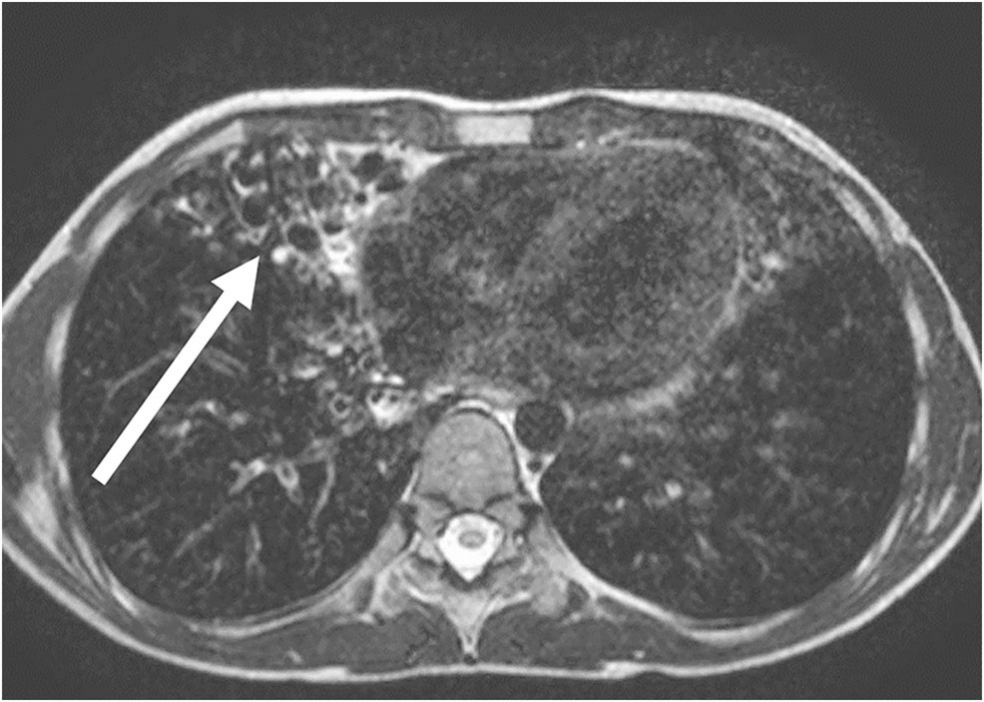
Lung MR in a 9-year-old girl with cystic fibrosis. Axial T2-weighted turbo spin-echo image (parameters in Table 2) shows multiple bronchiectases (*arrow*) in the right middle lobe. The bronchiectases are recognizable from the larger diameter and the thickened bronchial wall in contrast to the signal-free arteries
